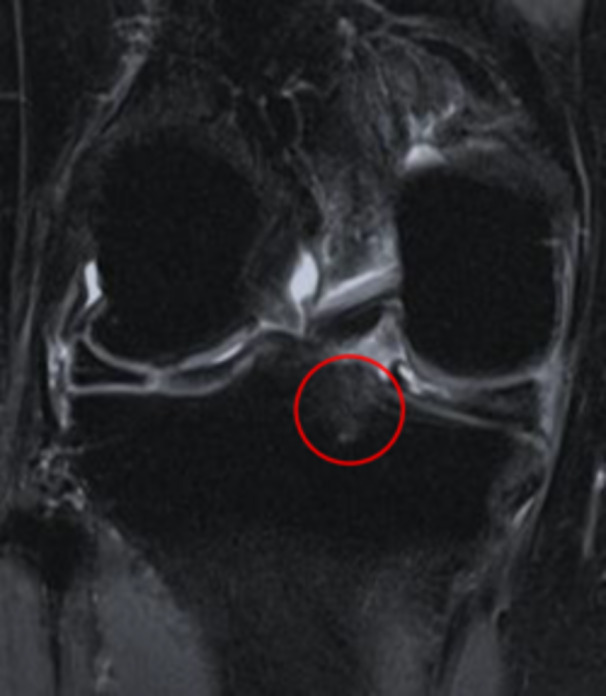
Magnetic resonance image of a posterior shiny‐corner lesion. Red circle, posterior shiny‐corner lesion.
